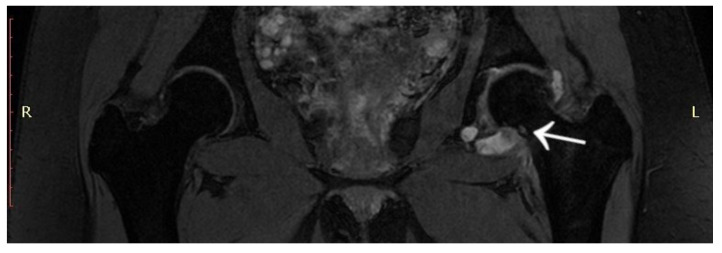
First MRI scan of the hip joints with visible focus of OO (arrow) in the femoral head/neck border, unnoticed.
